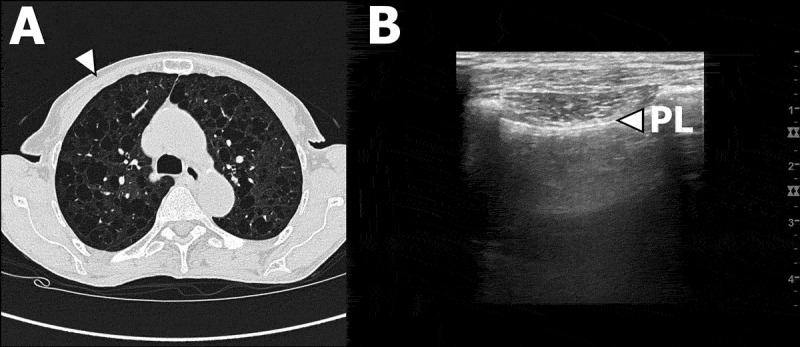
Patient #5 with LAM. (A) Transverse high-resolution computed tomography (HRCT) image of right and left upper and central lobe areas according to Belmaati.[26] White arrow corresponds anatomically to LUS zone 1 in B. (B) LUS clip of right LUS zone 1. White arrow indicates the pleural line.
